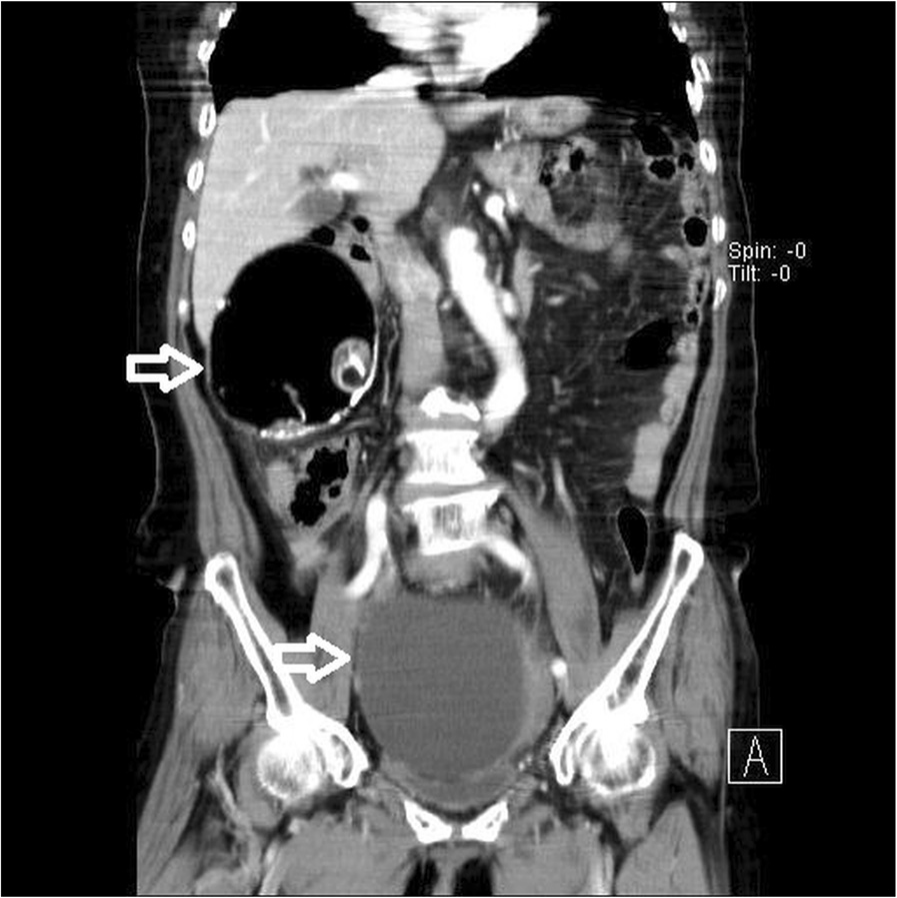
Abdominal computed tomography findings. The imaging indicates a mature cystic teratoma of the left ovary and right subhepatic space
